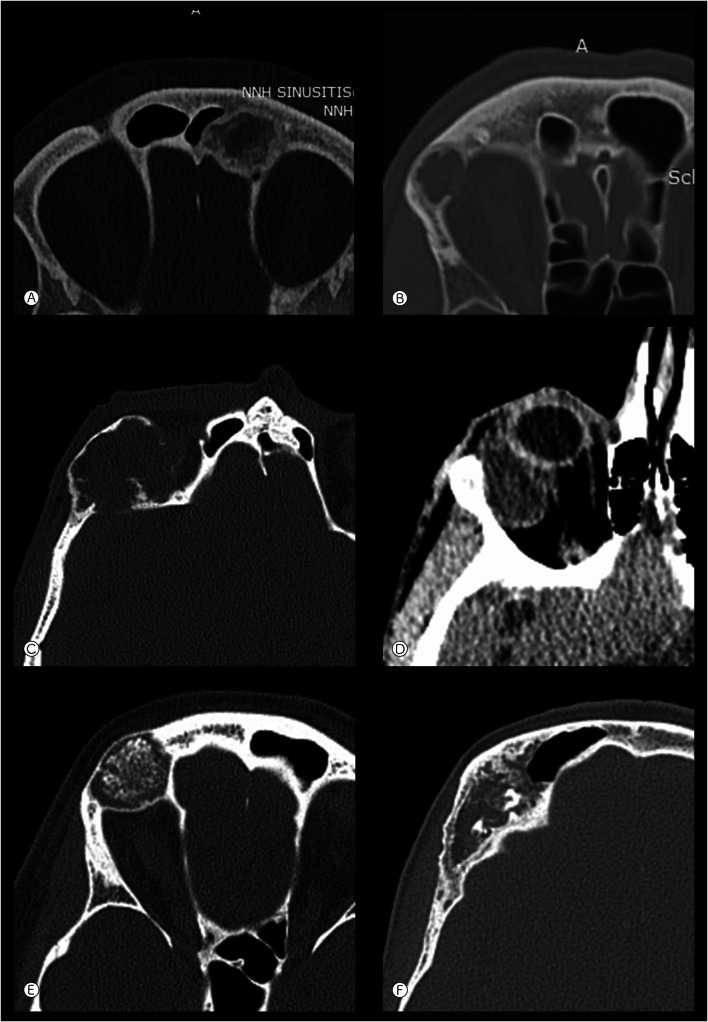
Frontal lesions. A Left frontal tumor expanding into the frontal sinus, showing thick peripheral sclerosis, within which is an irregular rim of increased density (case 7). B Right frontal tumor with mild bone expansion, a small focus of cortical destruction abutting the orbit and faint amorphous calcification (case 6). C and D Right frontal tumor with cortical destruction (C bone window), and, on a more inferior section, a mass in the orbit (D soft tissue windows) (case 4). E Right frontal tumor showing marked expansile remodeling and punctate mineralization (case 1). F Right frontal tumor containing irregular and curvilinear calcification (case 2) (all axial CT)
